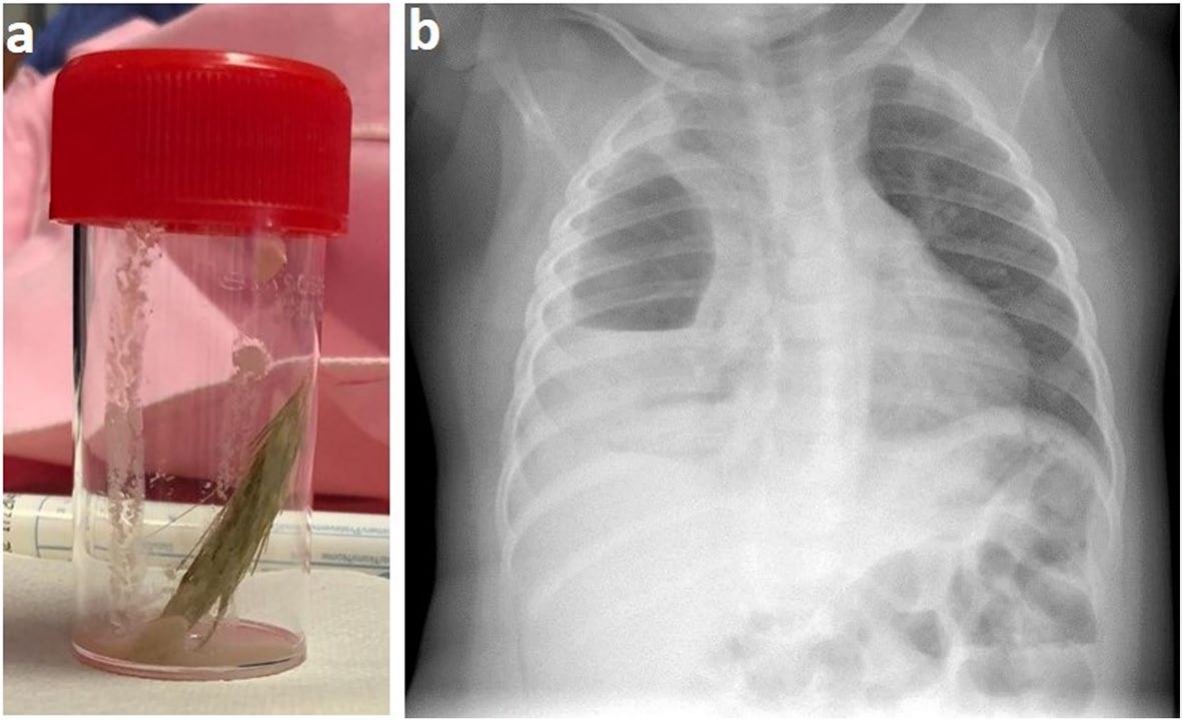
After the externalization: picture of the aspired wheat spikelet (2a); the chest X-ray shows a right pleural effusion (2b)
